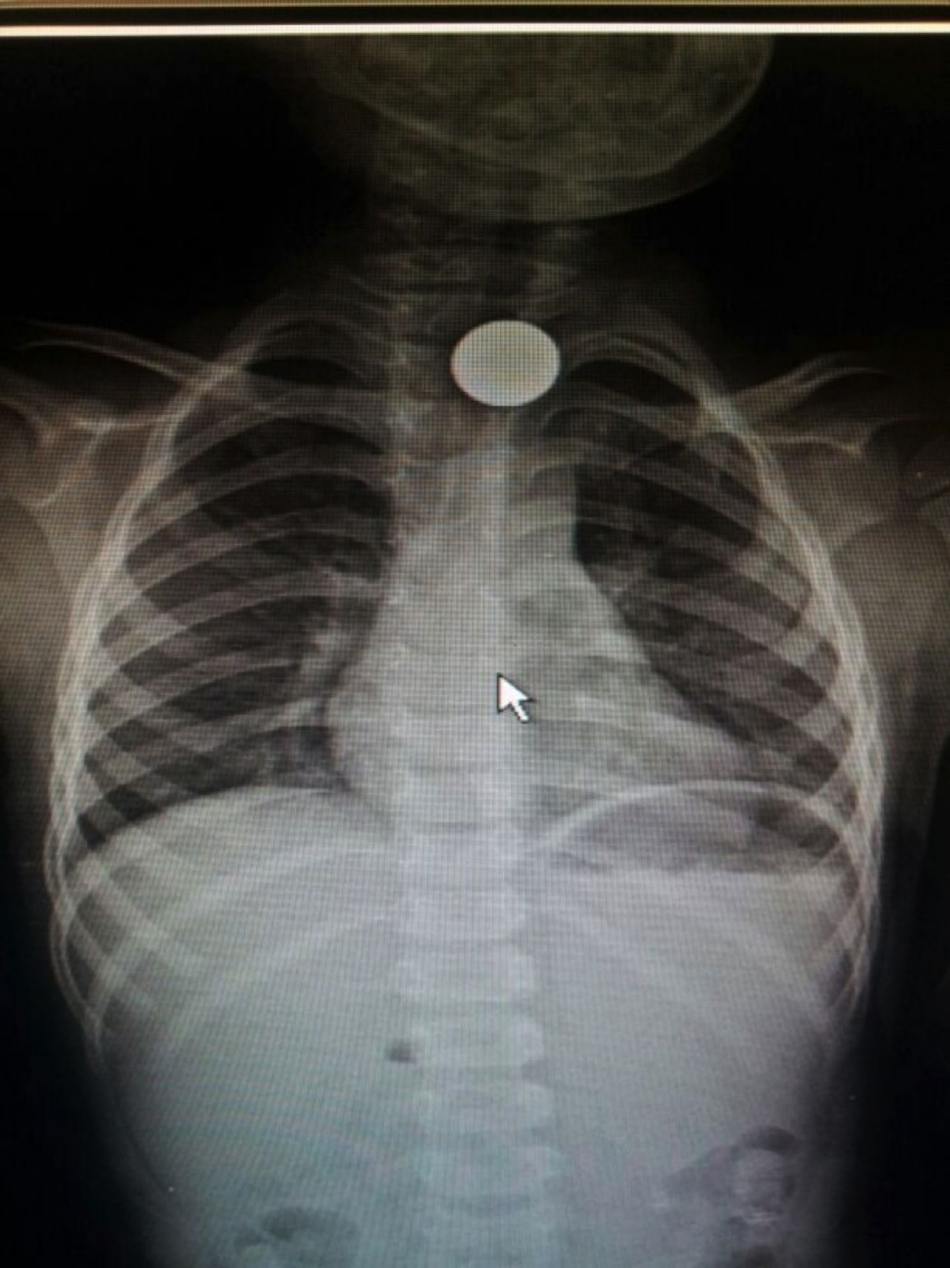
Chest X-ray demonstrating coin in the upper esophagus
